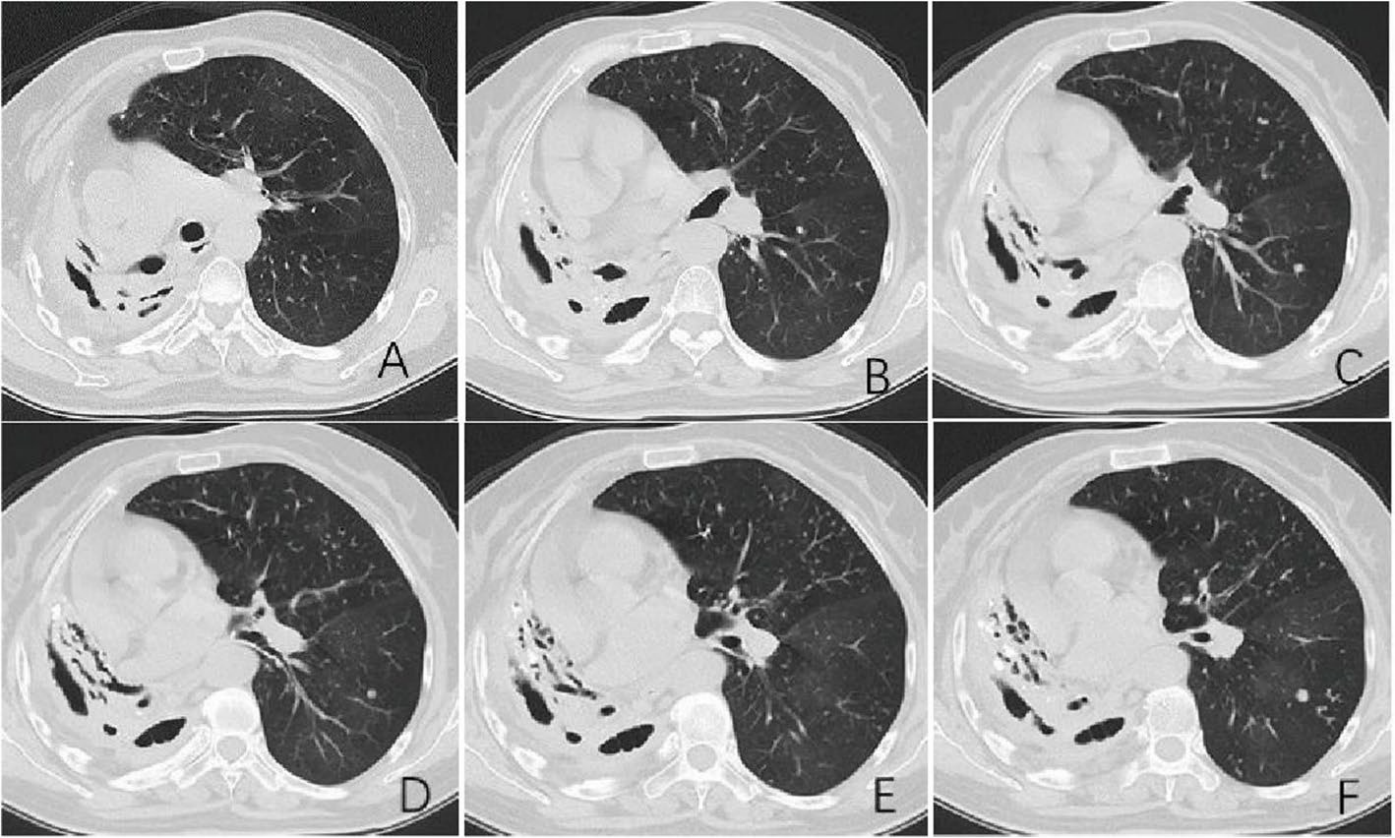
Representative chest CT findings in a 67-year-old female with DR-TB. Key features include: (i) destroyed right lung with segmental consolidation and air bronchograms; (ii) worm-eaten cavities and calcifications; and (iii) left-sided emphysema
